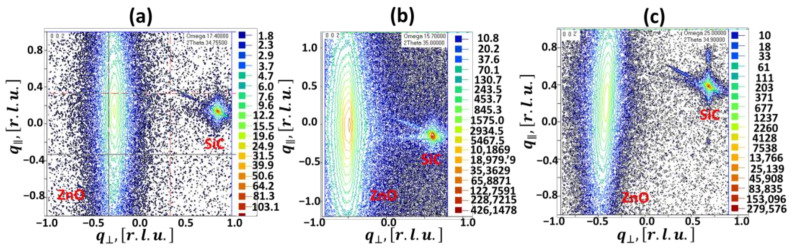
X-ray reciprocal space maps (RSMs) around the (002) reflection measured for ZnO films grown on SiC substrates with different off-cut angles: 0° (a), 2° (b), and 8° (c), respectively. Note: A 2° off-axis SiC substrate is chosen as a representative of SiC with low off-axis orientation, while 8^°^ off-axis SiC is regarded as a highly misoriented substrate. [r.l.u.] is reciprocal lattice units. Small spots correspond to SiC substrates. $q_{⊥}$ is the path in the *q*-space for the 2*θ*-*ω* scan, whereas $q_{∥}$ is the path in the *q*-space for rocking curve scan.
